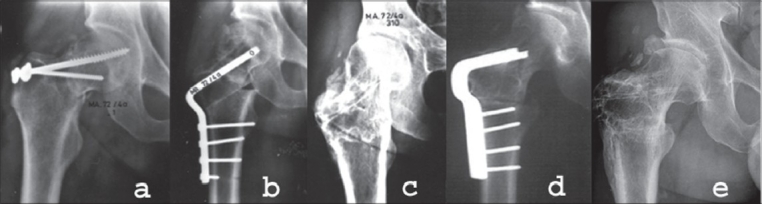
Secondary varization because of osteotomy in too much valgus: (a) AP x-ray in a 26 years male shows 8 weeks old fixation for femoral neck fracture. (b) Pauwels valgization osteotomy was performed in too much of valgus. (c) Six years followup x-ray of the same patient after implant removal shows signs of osteoarthrosis hip and patient was symptomatic. (d) Postoperative x-ray shows intertrochanteric varus osteotomy. (e) Ten years after last operation. The patient has mild pain and patient can walk with cane for 45 minutes, Harris hip score 79b. Too little bone stock in femoral head: If the bone stock in femoral head is less then the chances of implant cutout increases [Figure 6].
